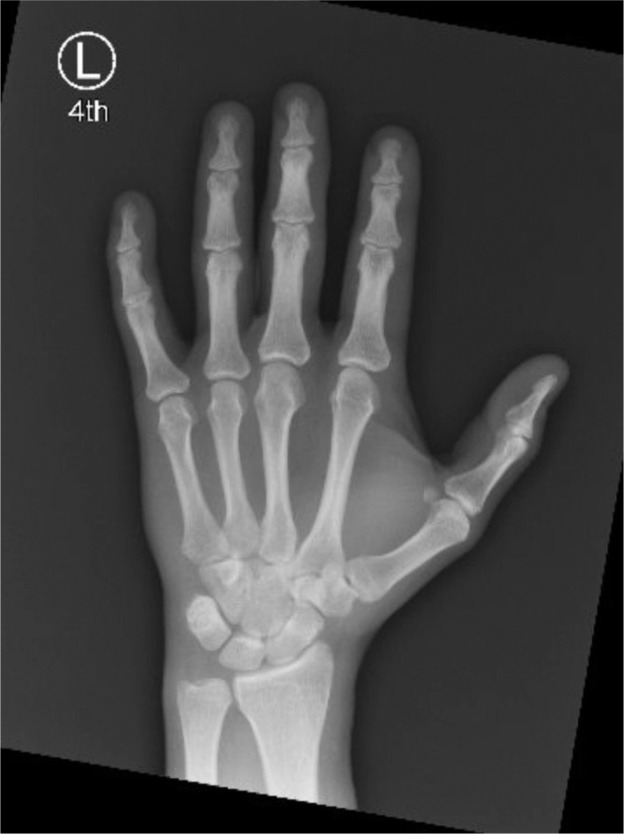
Frontal X-Ray of Left hand (Case 2: Pre-op).
